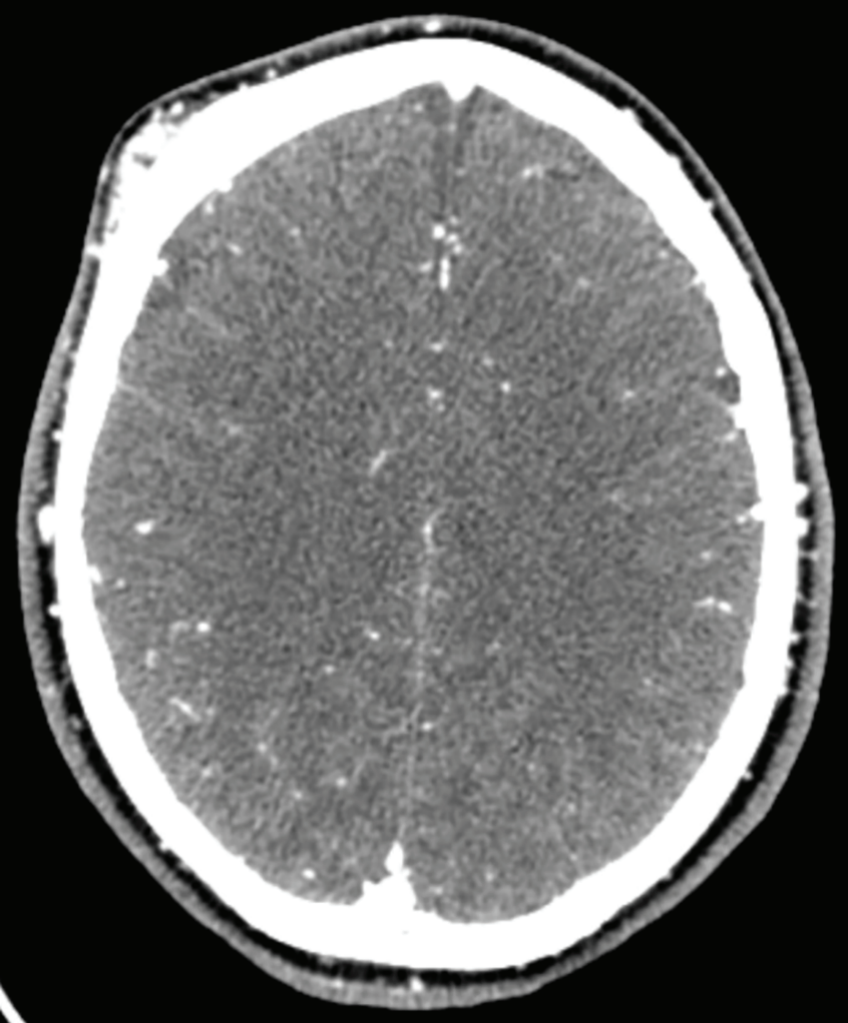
Brain CT-scan demonstrating arteriovenous malformation on the scalp
